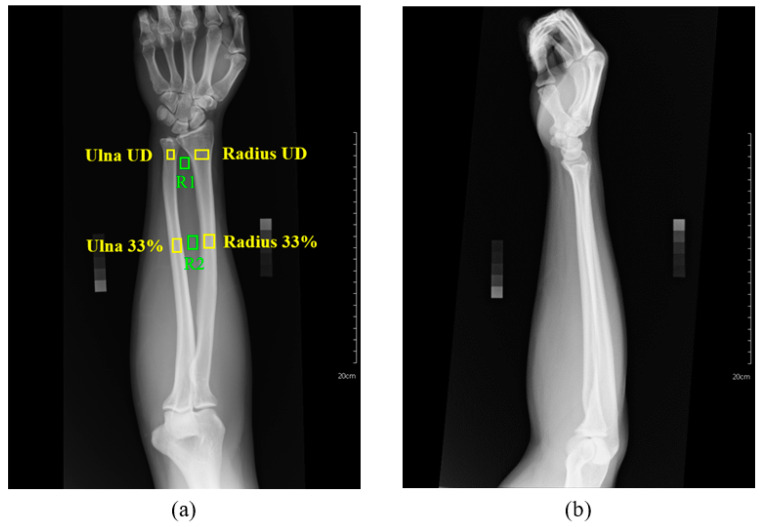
Different views of an adult forearm radiograph. (a) anterior-posterior view. Yellow rectangles represent different ROIs. Green rectangles represent the regions for estimating the average brightness of the soft tissues. (b) lateral view. Radius UD: ultra distal radius; Ulna UD: ultra distal ulna.
